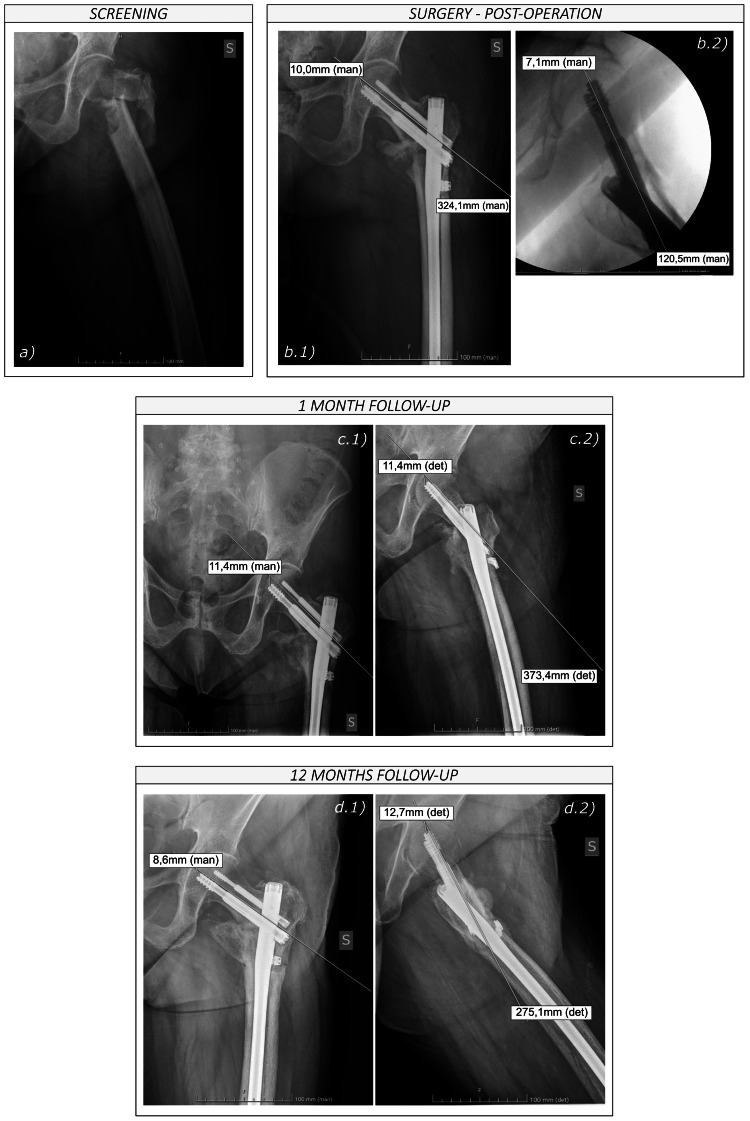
Radiographic course of a 72-year-old woman who sustained a low-energy left proximal femur fracture classified as AO/OTA 31-A2.3, comminuted and unstable. a) Preoperative anteroposterior radiograph obtained at screening. b.1-b.2) Immediate postoperative anteroposterior and lateral fluoroscopic images after fixation with a cephalomedullary nail with a 125° CCD angle and 380-mm nail length. The tip-to-apex distance (TAD) at surgery was 17.85 mm, calculated using the anteroposterior and lateral components shown on fluoroscopy (XAP = 10 mm; XLAT = 7 mm, automatically rounded by measurement software). c.1-c.2) One-month follow-up radiographs showing maintained implant position and early bone union; TAD was 23.1 mm. At this visit, the patient reported pain in the fractured hip. d.1-d.2) Twelve-month follow-up radiographs confirming fracture union and stable fixation; TAD remained 23.1 mm. The patient reported no hip pain at the second and final follow-up visits. No adverse events were recorded. AO/OTA: AO Foundation/Orthopaedic Trauma Association
